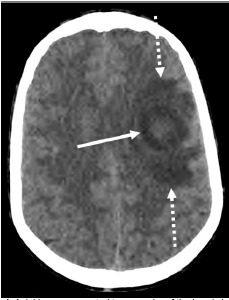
Axial image computed tomography of the head shows a rounded abnormality with mixed attenuation (solid arrow) in the left frontal lobe with surrounding hypodense vasogenic edema (dashed arrows).
